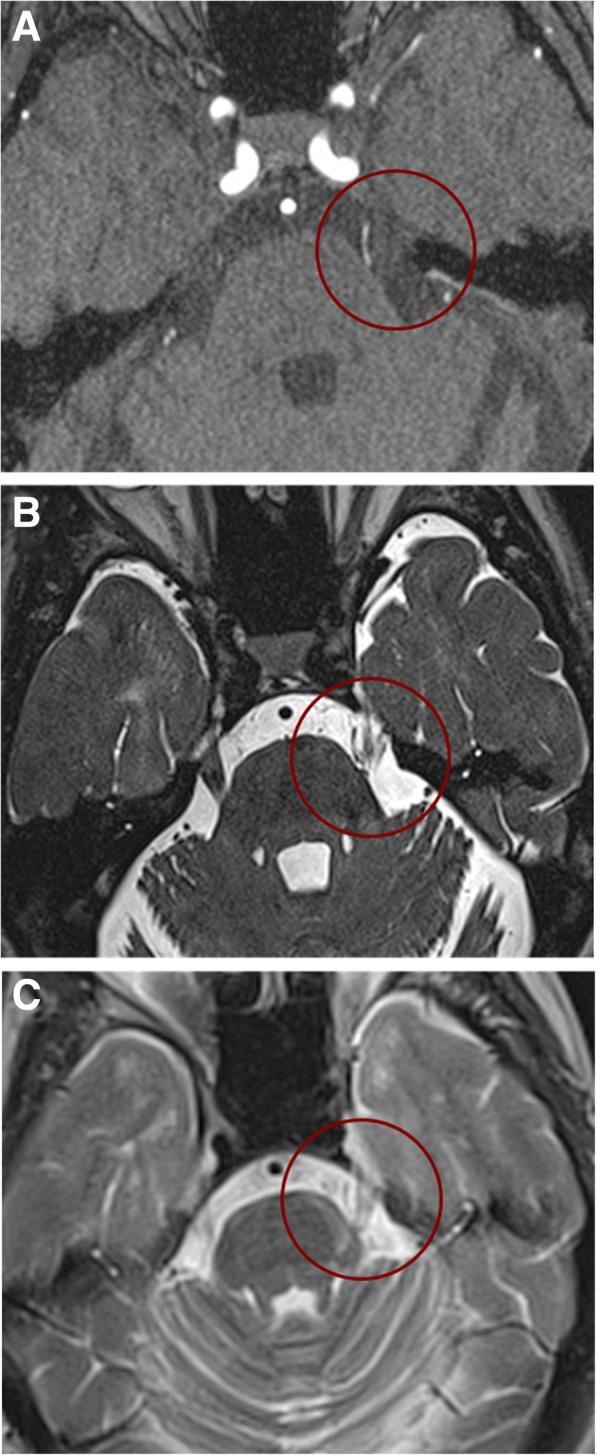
Neuroimaging findings in a representative patient with TN secondary to MS possibly due to a double crush mechanism. 3D time-of-flight (TOF) magnetic resonance angiography scans (a) and 3D constructive interference in the steady state (CISS) T2-weighted (b) on the axial plane demonstrate left neurovascular compression (NVC) with associated trigeminal nerve atrophy. T2-weighted image on the axial plane shows a hyperintense pontine lesion at the left trigeminal nerve root entry zone (REZ) (c). The arrow indicates the trigeminal nerve (b) and the arrowhead the demyelinating plaque (c). Reproduced from [16]
